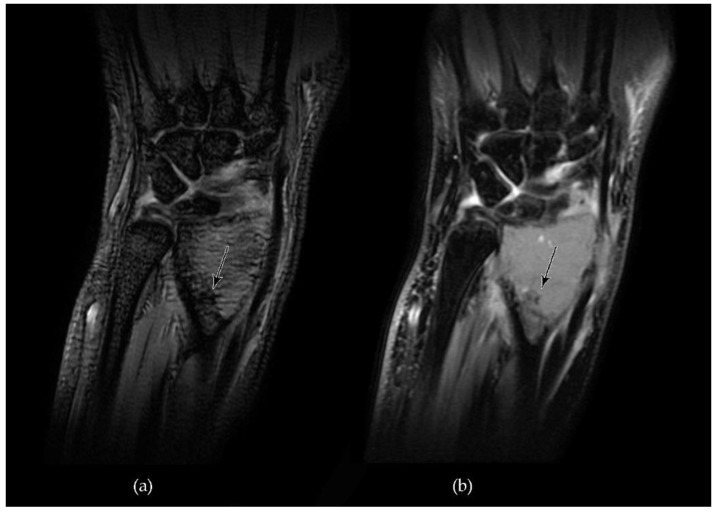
MRI appearance of hemosiderin deposits (arrows) in a case of GCTB of the left radial metaepiphysis (a). T2*-W GRE sequence demonstrates a markedly and distinctly hypointense area that appears more tenuously hypointense in a T2-W Fast Spin Echo (FSE) sequence (b).
